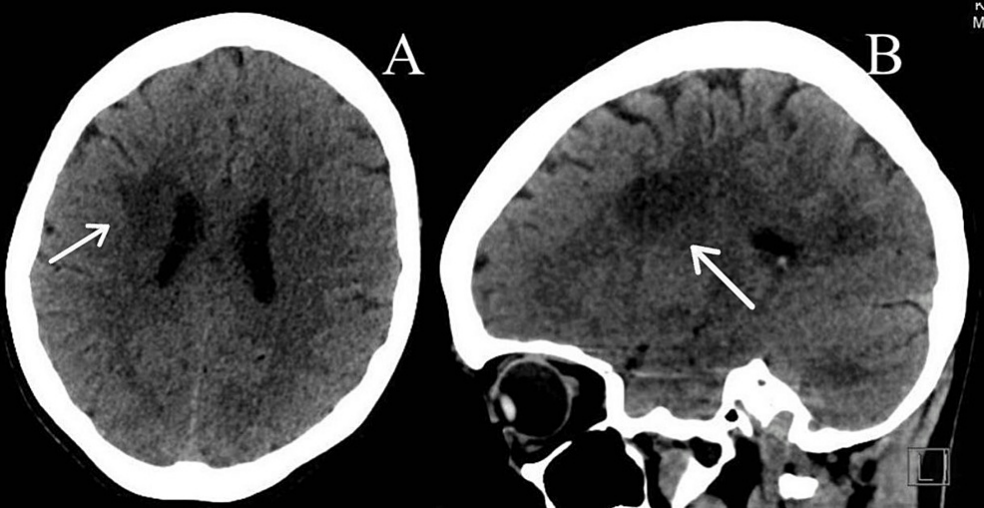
Brain CT (A) axial and (B) sagittal images show large right periventricular hypodensity.
